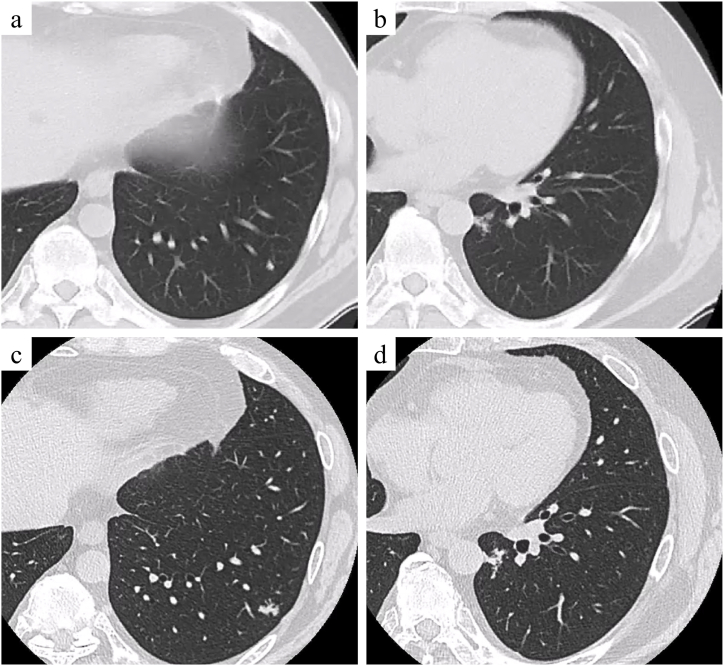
Radiological findings. a, b: Computed tomography showing nodules in segments S9 and S10 of the left lung, first detected after surgery for colon cancer (a: S9, b: S10). c, d: Nodules showing linear and branching opacities described as a tree-in-bud pattern, 15 months following initial nodule detection (c: S9, d: S10).
